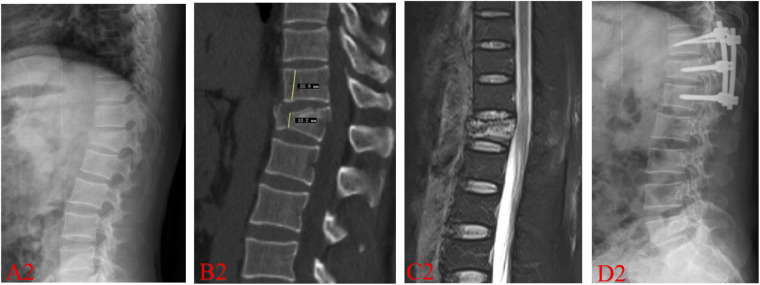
Representative case 2. A 50-year-old male presented following a motor vehicle accident. CT imaging (B2) revealed a T12 burst fracture characterized by severe comminution of both the superior and inferior endplates and vertebral height collapse exceeding 50%, while spinal canal encroachment remained less than 50%. The posterior ligamentous complex (PLC) appeared intact, and no neurological deficits were identified. MRI (C2) indicated mild intervertebral disc injury. The patient received a TLICS score of 2 (indicating conservative treatment), an NmTLICS score of 4 (indicating an indeterminate recommendation), and a CN-mTLICS score of 3 (indicating conservative treatment). Ultimately, the patient underwent posterior spinal fixation from T11 to L1 (D2). In this instance, both TLICS and CN-mTLICS failed to align with the actual treatment regimen, whereas only NmTLICS successfully predicted the surgical intervention. CT, Computed Tomography; MRI, Magnetic Resonance Imaging; TLICS, Thoracolumbar Injury Classification and Severity Score; NmTLICS, Novel Modified Thoracolumbar Injury Classification System; CN-mTLICS, China-modified Thoracolumbar Injury Classification System.
